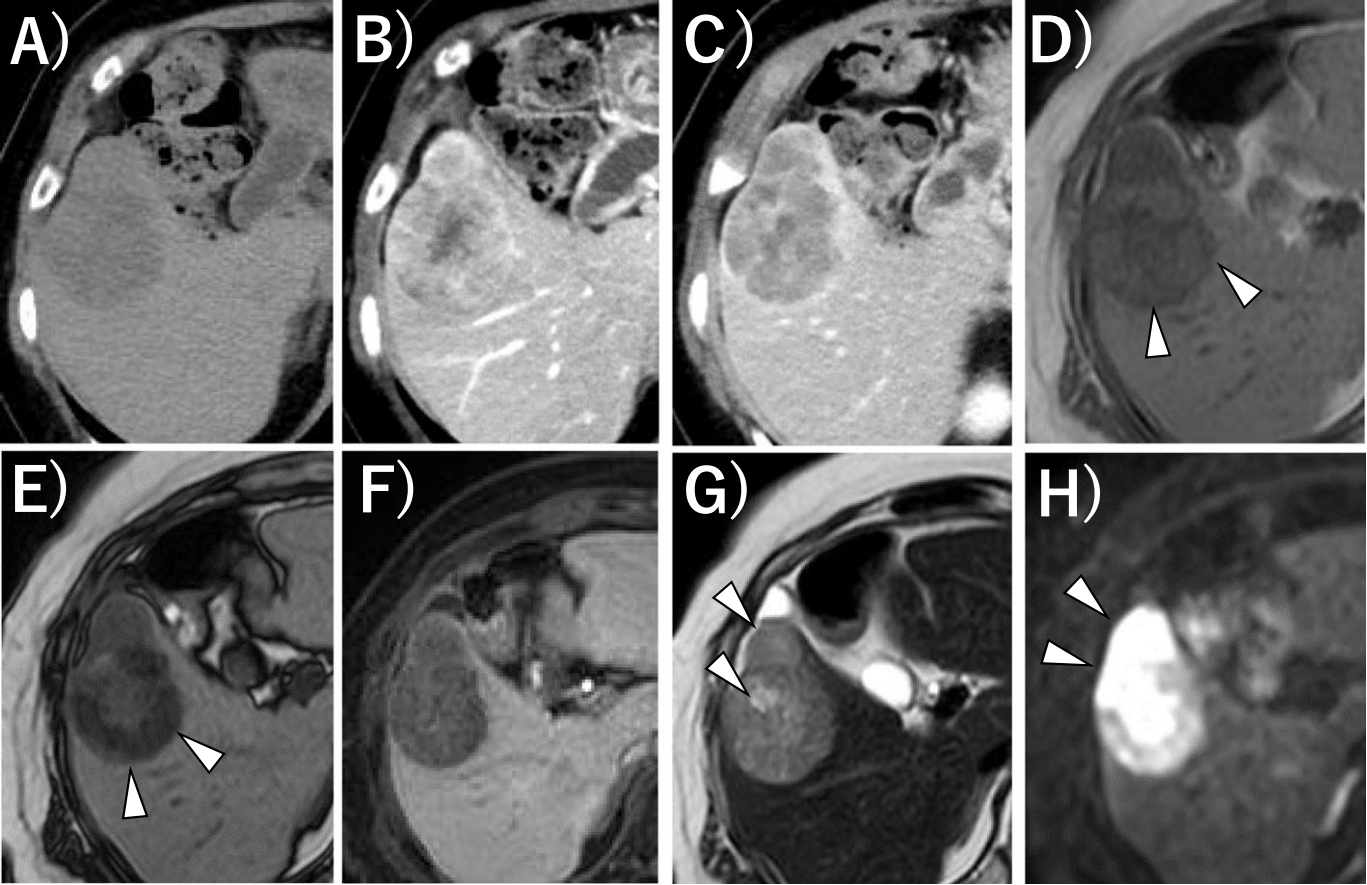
Preoperative CE-CT and EOB-MRI images. a Plain CT of the liver showed a 48×43 mm low-density lesion in the liver. b, c CE-CT showed contrast enhancement in the arterial phase (b) and hypoattenuation in the delayed phase (c). d–h EOB-MRI of the liver. The lower and peripheral parts of the lesion showed a lower signal in T1 weighted out-of-phase images (e) compared with in-phase images (d) (arrowhead). The lesion showed a low signal in the hepatobiliary phase (f). Fat-suppressed T2-weighted image (g) and diffusion-weighted image (h) showed a high signal in the upper and central parts of the lesion (arrowhead)
